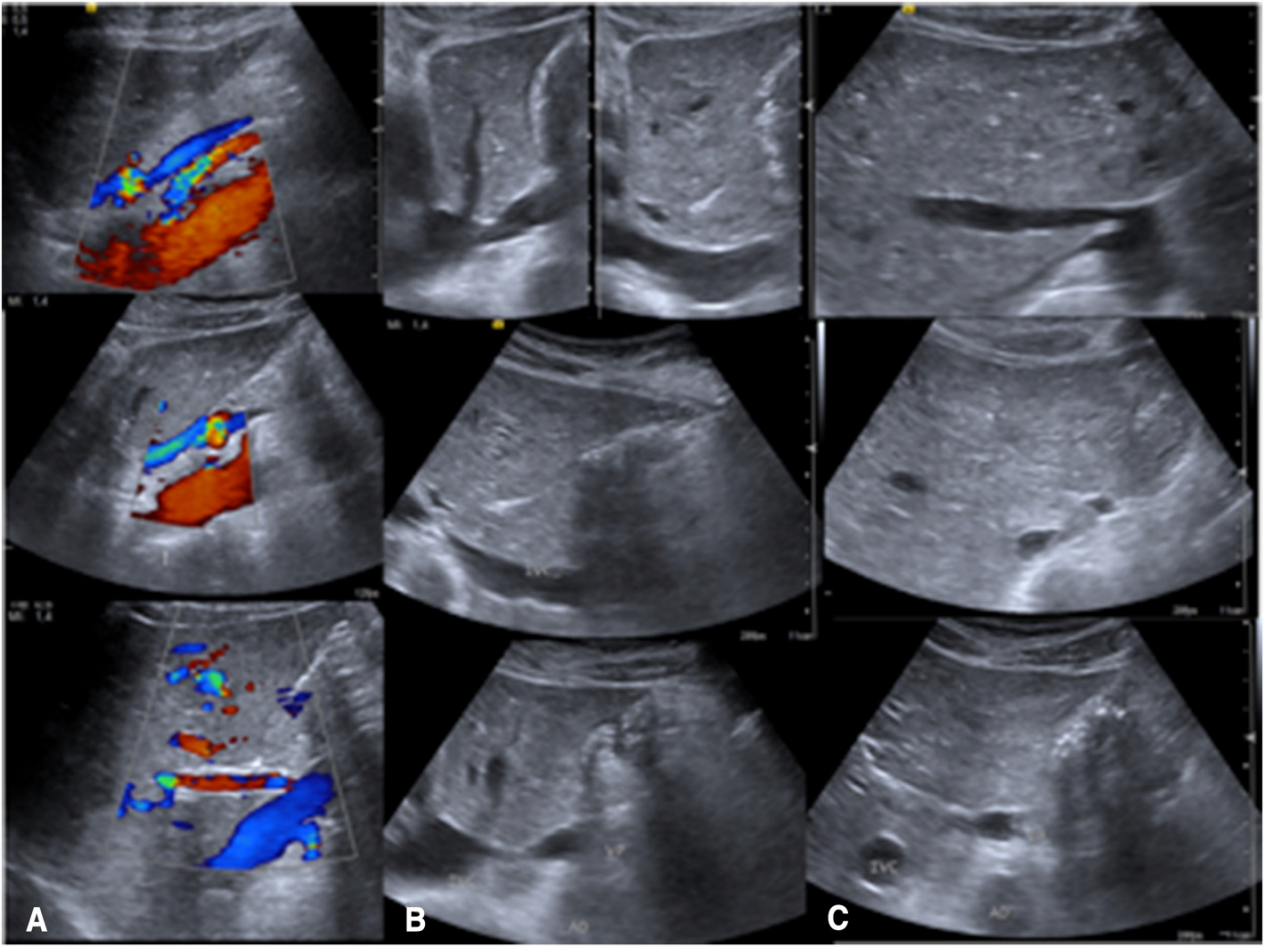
Color Doppler Ultrasound with sagittal and axial planes on case 2 (Type 2). Note: a: The abdominal aorta, mesenteric artery, extension of mesenteric vein and also the extrahepatic portal vein that directs to the head and posterior region of the lateral segment. At hepatic hilum level there is an evidence of the inferior vena cava and an increased caliber of hepatic artery. b: The sagittal and paramedian planes show two retro hepatics paths of the portal extrahepatic vein (along the venous ligament). c: Ultrasound in axial plane, in the level of the confluence of the hepatic veins to IVC and cross-section of the inferior vena cava, the extrahepatic portal vein door and the abdominal aorta
